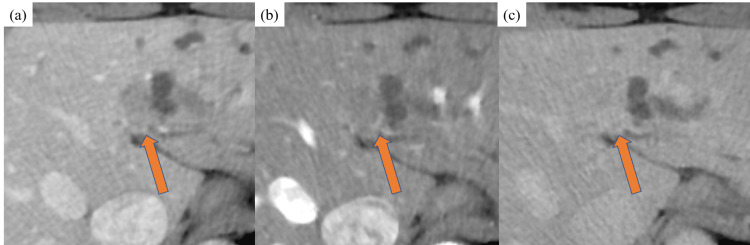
Contrast-enhanced CT images of a 74-year-old man with 20-mm perihilar bile duct cholangiocarcinoma (arrow) (a) Axial portal phase showing a low-density mass with the left primary and secondary bile duct; B2 and B3 bile ducts located upstream of the tumor are dilated. The mass extended beyond the bile duct and the parenchyma of the liver. (b) and (c) Axial arterial and delayed phases showing the mass demonstrated non-notable contrast enhancement compared with the liver parenchyma.
